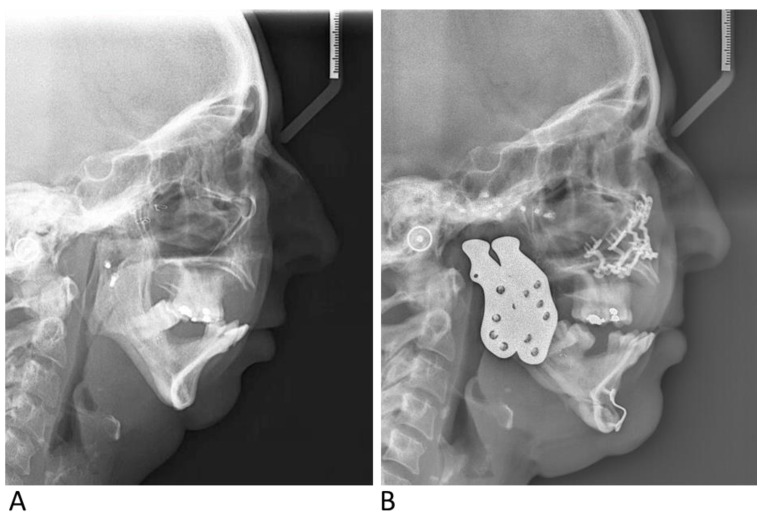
Preoperative (A) and postoperative (B) lateral cephalometric radiographs of case 2.
